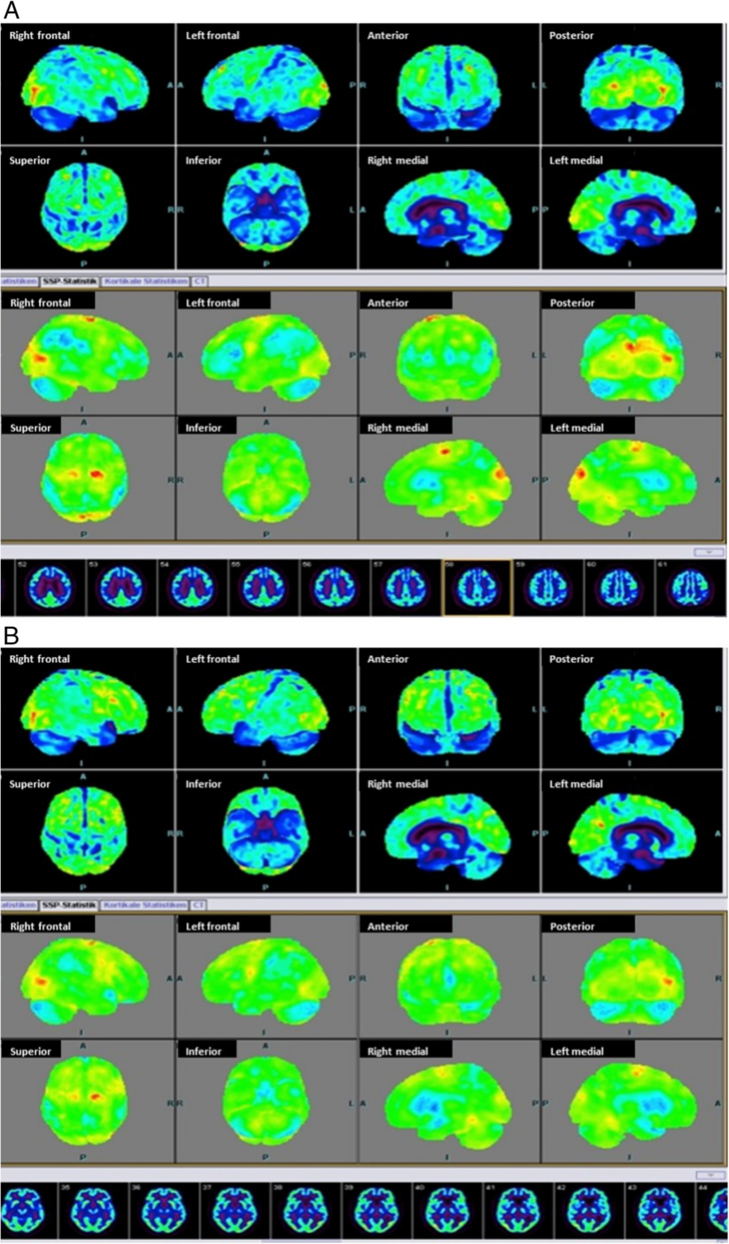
FDG-PET on a May,23rd, with an Alzheimer like glucose utilization deficit in temporo-parietal regions and the posterior cingulum and b June, 25th, with a normalization of glucose utilization in all brain regions
